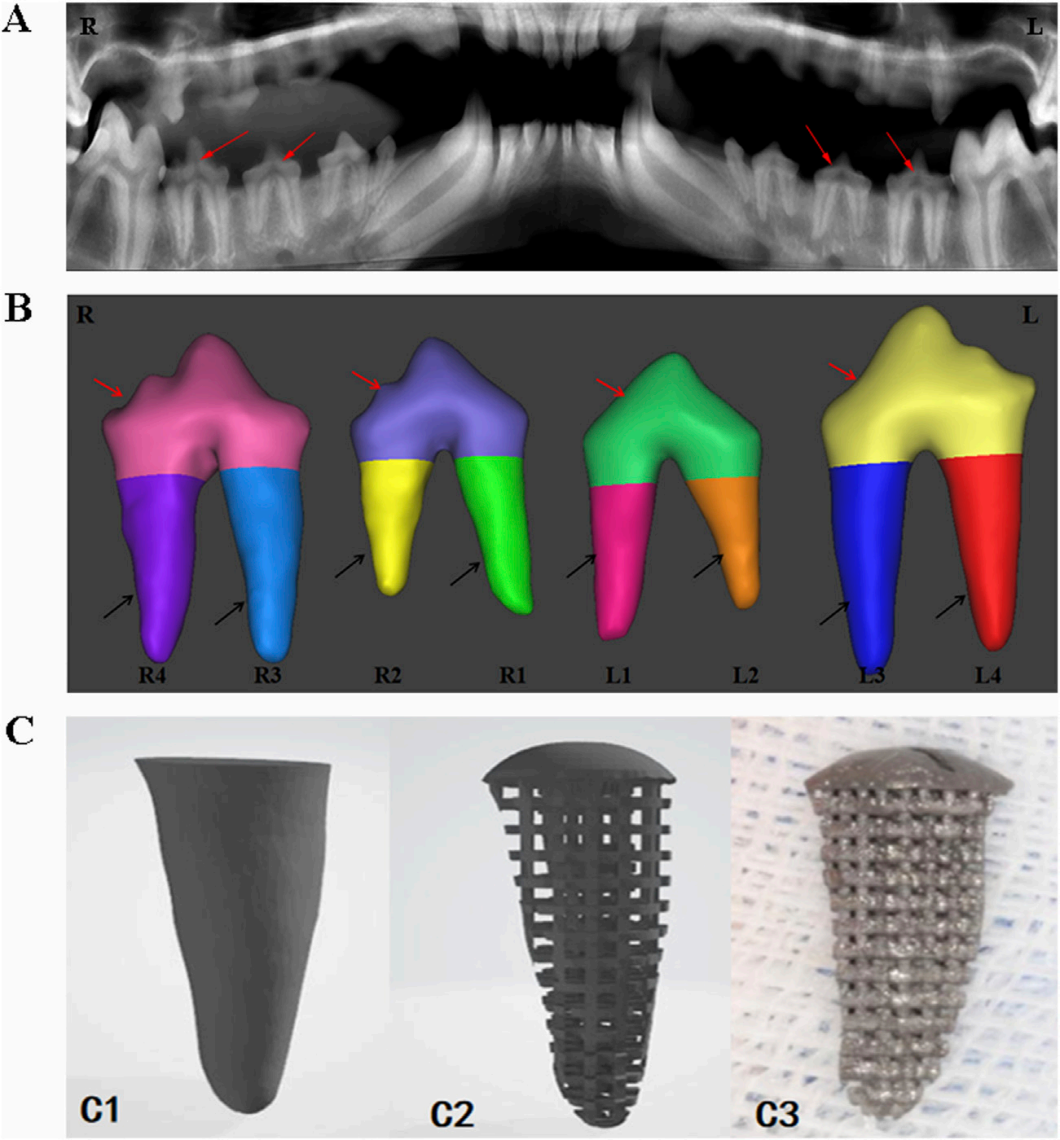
Digital model acquisition, design, and printing of RAIs. (A) CBCT data acquisition. (B) 3D model of target tooth, the red arrow indicates the crown part, and the black arrow indicates the root part. (C1) Extract the target tooth root image. (C2) Constructing a three-dimensional digital model of porous structures. (C3) 3D printing of porous titanium RAIs that match the shape of the target tooth root.
